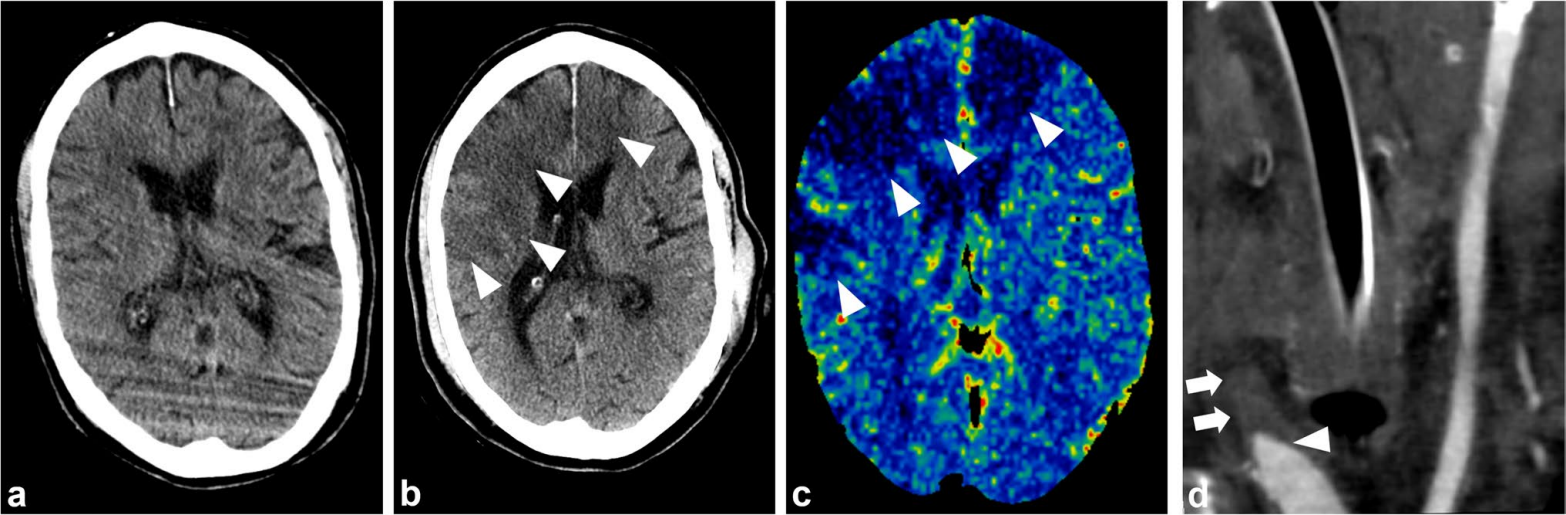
Patient aged 68 with ankylosis caused by degenerative spondylosis from the fifth to seventh cervical vertebrae. While moving with a walker, he was hit by a car going at 45 km/h, which inflicted fractures of the dens axis, ribs, and thoracic spine, in addition to multiple pelvic ring fractures. Following routine blunt trauma protocol, a non-enhanced head CT was obtained, which showed no signs of acute TBI or ischemia (a). Endovascular embolization of the right internal iliac artery stopped active extraperitoneal bleeding four hours after admission. Eight hours after admission, the patient fell unconscious, and an extensive acute infarction appeared in the right medial and both anterior cerebral arteries’ vascular territories (arrowheads) in a non-contrast head CT scan with 3 mm transverse slices (b) and on blood flow image of contrast-enhanced perfusion CT (c). A coronal reformatted image of 2 mm thickness from a CTA shows total occlusion of the right common carotid artery by a Biffl grade IV BCVI (arrowhead), distally to which the lumen shows no enhancement (arrows) (d). BCVI, blunt cerebrovascular injury; CTA, CT angiography
